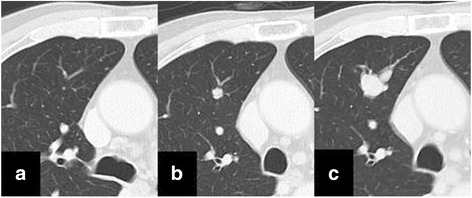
Chest computed tomography. There was no tumor shadow in the lung when the resection for rectal cancer was performed (a). A solid nodule in the right upper lung lobe was first detected 18 months after surgery (b). The nodule grows in diameter 6 months later (c)
